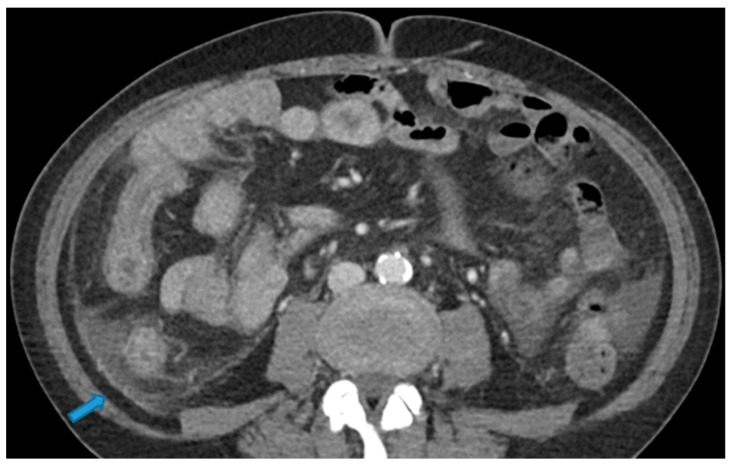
Axial CE-CT. Observe the enhancing pseudonodular parietal peritoneum (arrows), which corresponds to collaterals vessels, and is not to be mistaken for peritoneal deposits.
